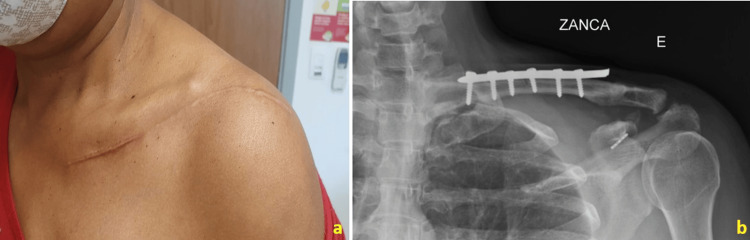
(a) Ectoscopic assessment of the patient at the six-month postoperative follow-up, demonstrating the postoperative appearance of the surgical incision with complete healing and no complications. (b) Radiographic control in the Zanca view of the left clavicle at nine months.
